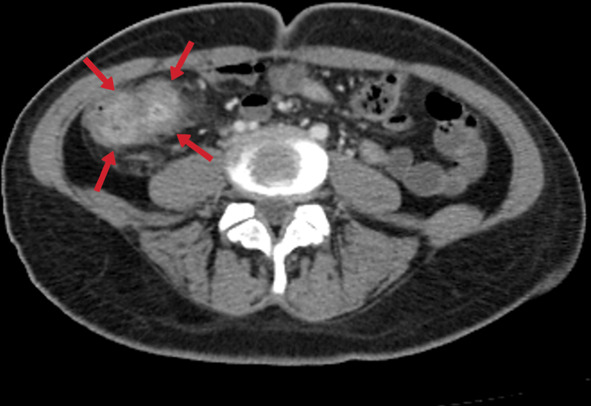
A 31-year-old female. Contrast-enhanced CT image demonstrates diffuse-symmetric wall thickening and enhancement of the cecum with surrounding inflammatory changes (arrows)
